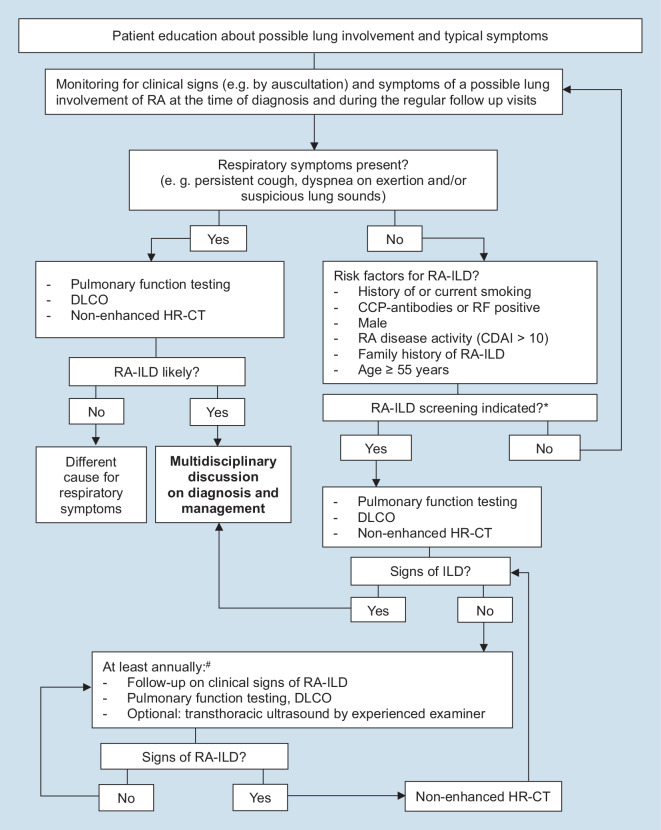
Suggested screening algorithm for rheumatoid arthritis-associated interstitial lung disease (*RA-ILD*) in incident patients with rheumatoid arthritis and follow-up after initial screening. *RA* rheumatoid arthritis, *DLCO* diffusion limitation for carbon monoxide, *anti-CCP* cyclic citrullinated peptide antibodies, *RF* rheumatoid factor, *HR-CT* high-resolution computed tomography, *CDAI* Clinical Disease Activity Index. *Asterisk* Quantity and significance of existing risk factors to trigger continuous RA-ILD screening cannot be given at the moment, and these are therefore at the discretion of the treating physician on a case-by-case decision. Rhombus Necessity of regular non-contrast HR-CT and diagnostic interval of examinations is at the discretion of the attending physician on the basis of a case-by-case decision
